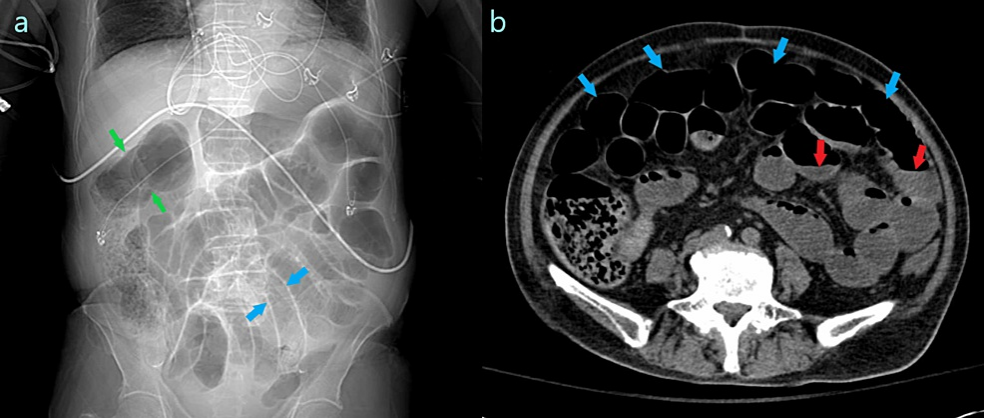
Computed tomography demonstrating ileus. (a) Scout image from computed tomography of the abdomen demonstrating diffuse gaseous distention of the large bowel (green arrows) and small bowel (blue arrows). (b) Axial computed tomography image through the abdomen demonstrating multiple loops of dilated small bowel (blue arrows) with air fluid levels (red arrows) and no transition point.
